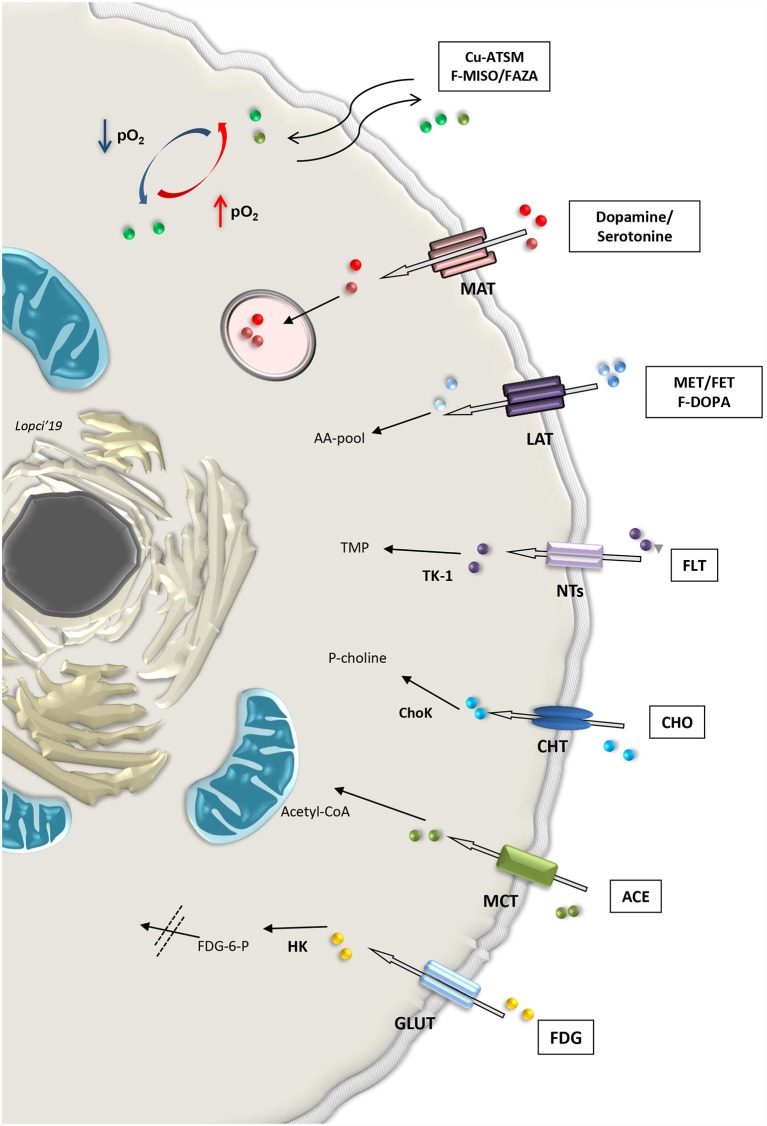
Summary of selected metabolic pathways detectable non-invasively with radiolabeled compounds applicable with PET imaging in clinical practice. Abbreviations: Cu-ATSM, Copper(II)-diacetyl-bis(N(4)-methylthiosemicarbazone; F-MISO, fluoromisonidazole; FAZA, Fluoroazomycin Arabinoside; MAT, monoamine transporter; MET, methionine; FET, fluoroethyltyrosine; LAT, large amino acid transporter; F-DOPA, fluoro-3,4-dihydroxyphenylalanine; AA-pool, amino acid pool; FLT, Fluorothymidine; NTs, nucleoside transporter families; TK1, thymidine kinase-1; TMP, thymidine monophosphate; CHO, choline; CHT, choline transporter; ChoK, choline kinase; P-choline, phosphocholine; ACE, acetate; MCT, monocarboxylate transporter; FDG, fluorodeoxyglucose; GLUT, glucose transporter; HK, hexokinase; FDG-6-P, fluorodeoxyglucose-6-phosphatase.
